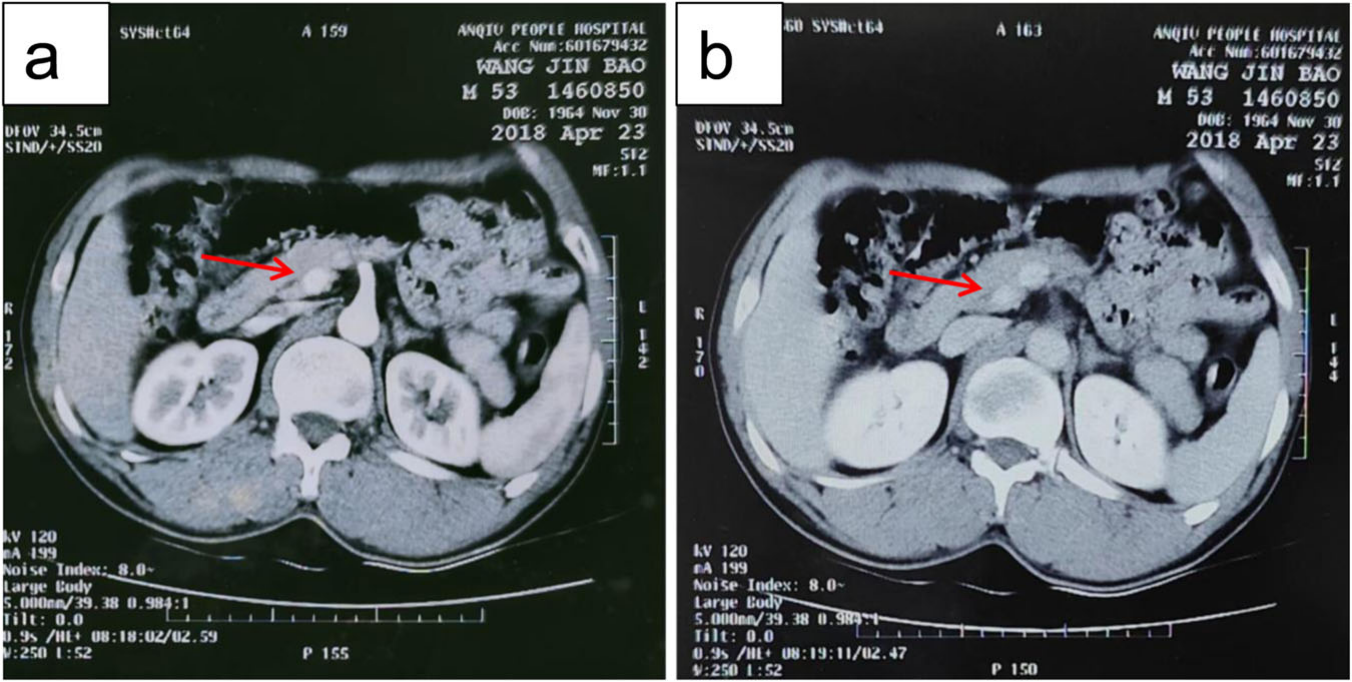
Abdomen enhanced CT scan of case 1. a, b Abdomen enhanced CT revealed a round, well-circumscribed, and arterialized lesion at the tail of the pancreas (arrow)
